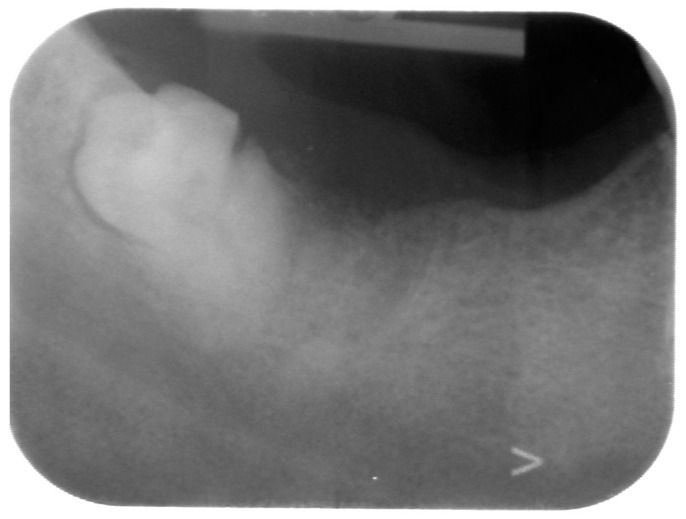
Intra oral X-ray showing a partly impacted fractured lower right wisdom tooth.
